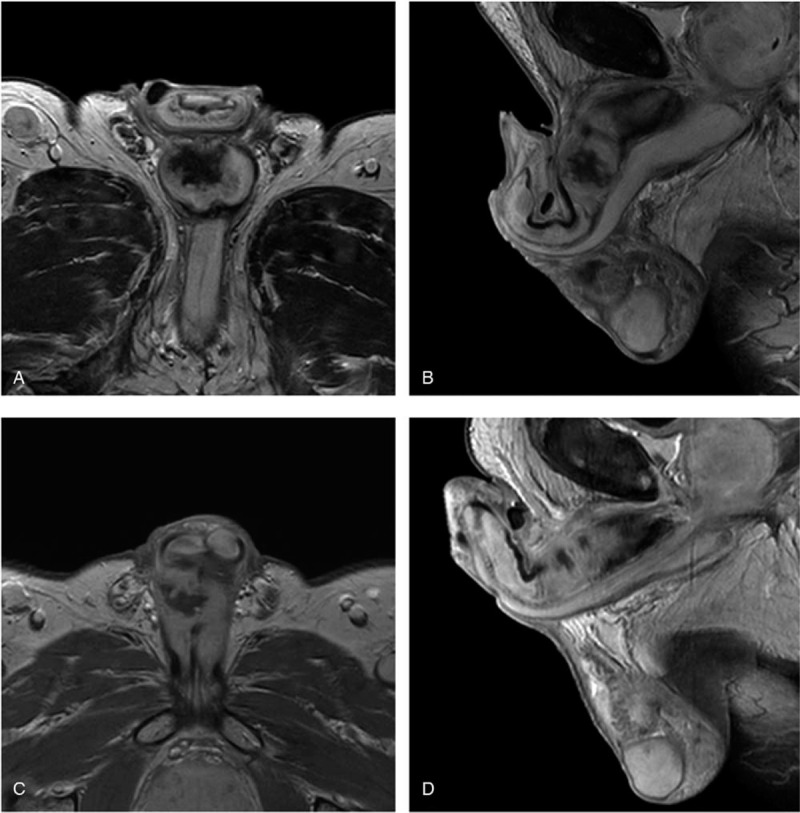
Gadolinium-enhanced fat-suppressed T1-weighted magnetic resonance imaging image showing a low intensity lesion. (A) Axial view before chemotherapy. (B) Sagittal view before chemotherapy. (C) Axial view after chemotherapy. (D) Sagittal view after chemotherapy.
